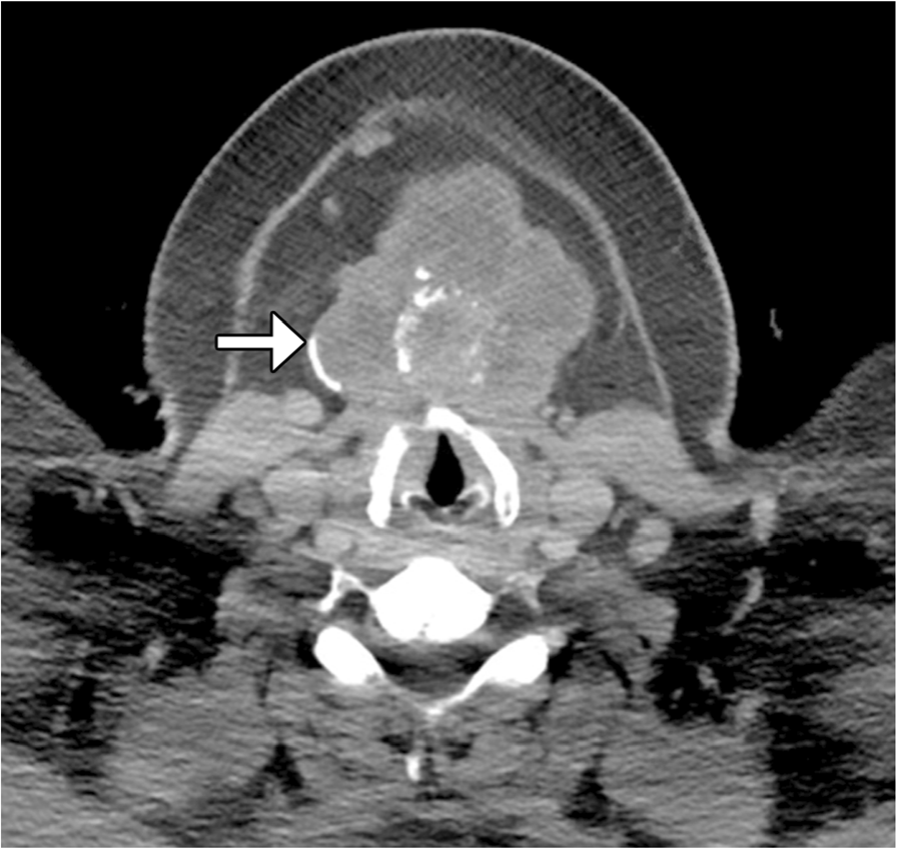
Papillary thyroid carcinoma arising from thyroglossal duct cyst. Axial CT image shows a bulky cystic and partly calcified mass in the midline anterior neck (arrow)
